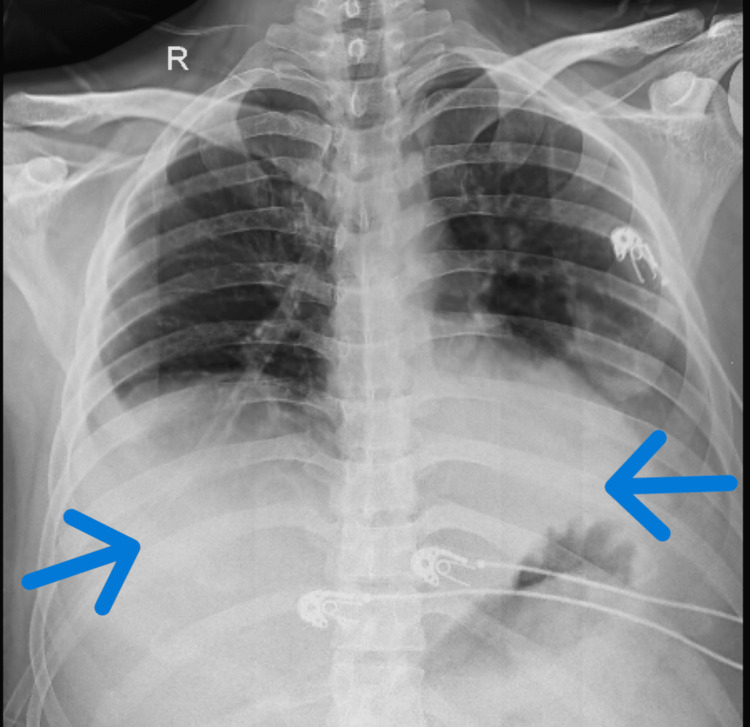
Radiograph (postero-anterior view) revealing pleural effusion on left > right side Homogeneous density is seen in lower zones, diminished cardio-phrenic and costophrenic angle. The accumulation of fluid highlighted with arrows at right and left lower zones.
